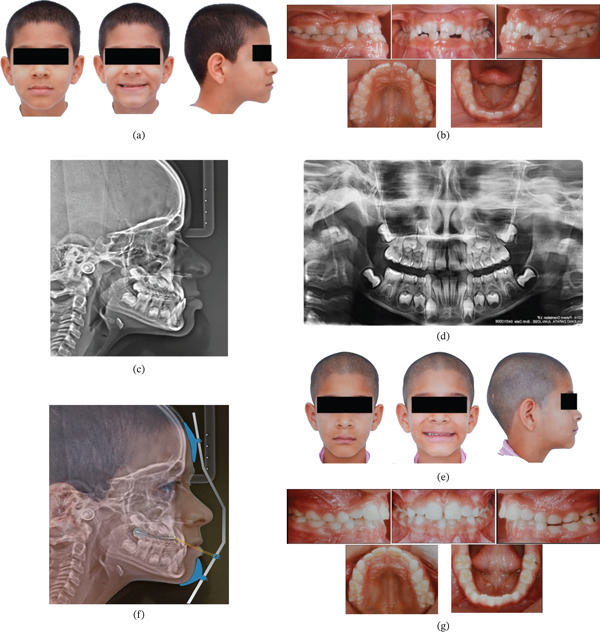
(a) Pretreatment facial photographs showing characteristic features of Class III malocclusion including mildly concave profile and deficient maxillary projection. (b) Pretreatment intraoral photographs demonstrating anterior and posterior crossbites, and Class III molar relationship. (c) Pretreatment lateral cephalometric radiograph revealing skeletal Class III pattern with maxillary deficiency and mandibular prognathism. (d) Pretreatment panoramic radiograph showing mixed dentition with all permanent teeth developing normally. (e) Clinical application of Petit‐type facemask with protraction elastics. (f) Facial photographs following maxillary expansion and protraction therapy showing improved facial balance. (g) Occlusal photographs after active orthopedic treatment demonstrating correction of crossbites.
